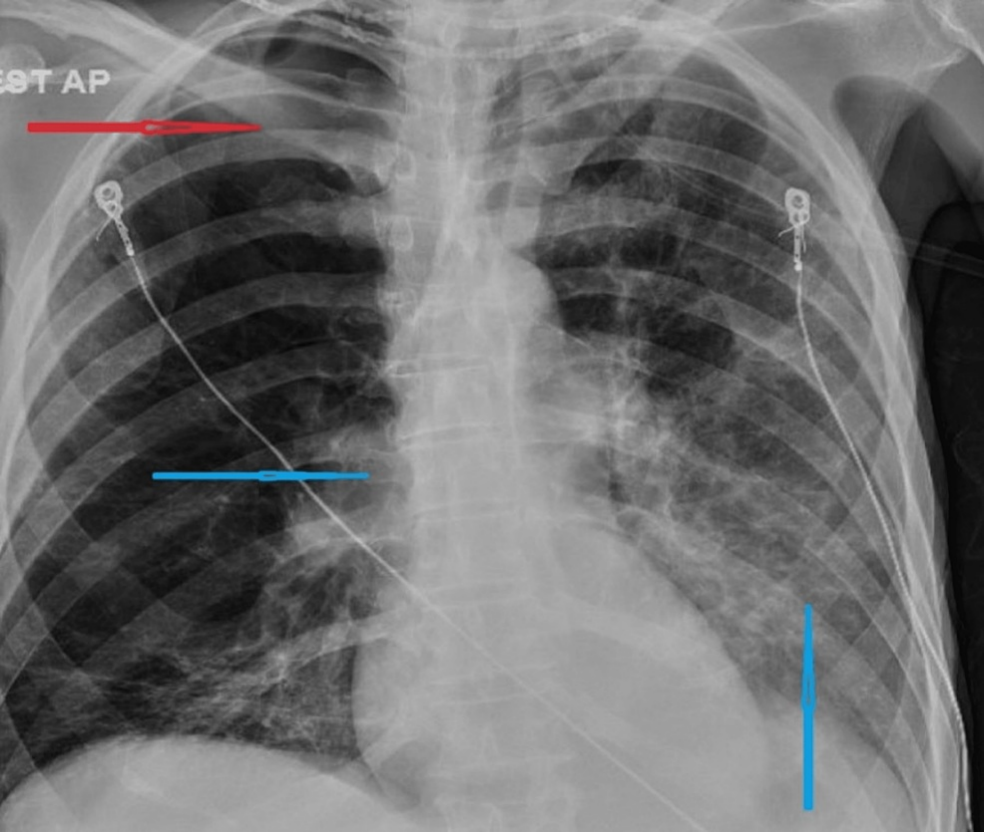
Chest X-ray at presentation showing left lower lobe and right infrahilar opacity (blue arrows) and right apical bullous change (red arrow)
